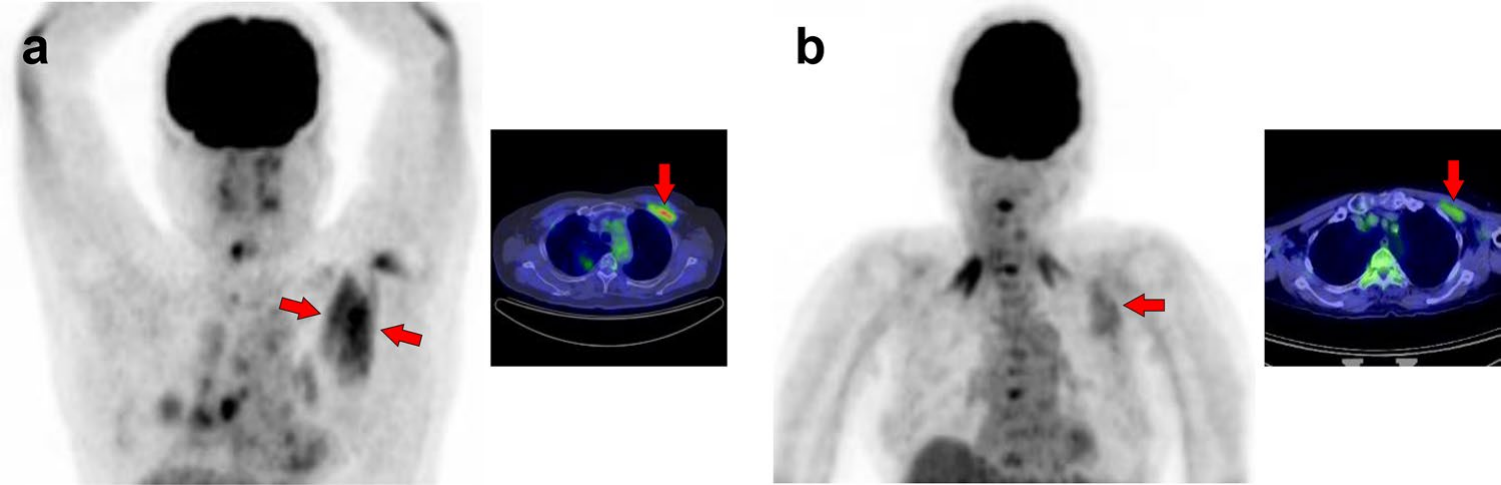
Pectoralis minor muscle.* The pectoralis minor can be observed without visualization of the pectoralis major (a, b, arrows). Conversely, uptake in the other muscles is occasionally accompanied, and we can find the uptake in the supraspinatus muscle in case (a) and in scalene muscle in case (b). CT should also be evaluated carefully when a cardiac pacemaker is implanted, as it can be confused with postoperative inflammation or attenuation correction errors due to CT metal artifacts
